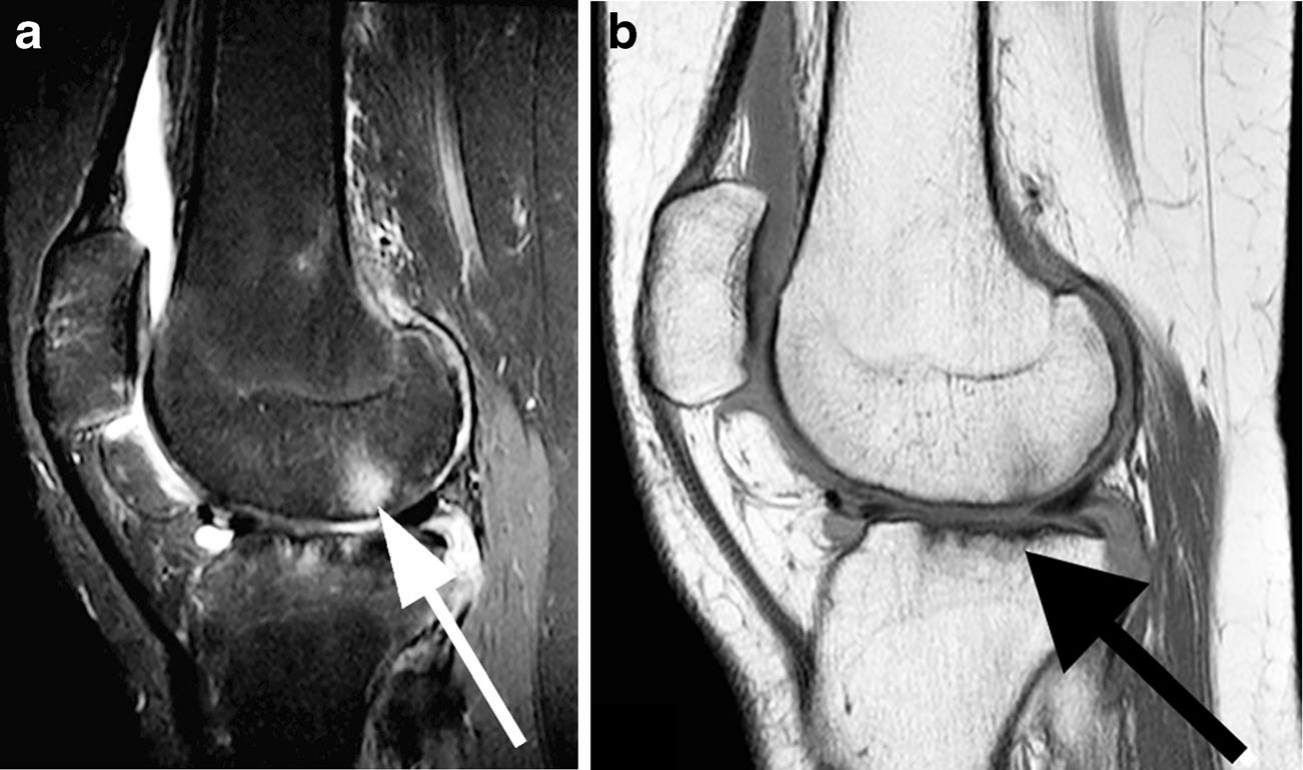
Sagittal MR images of the knee in a 16-year-old girl with poly-articular juvenile idiopathic arthritis. a T2-weighted sagittal MRI with fat suppression shows bone marrow oedema in the femur (*arrow*) and tibial plateau. b T1-weighted sagittal MRI shows bone erosions in the tibial plateau (*arrow*), with irregular signal intensity of the cortical bone and loss of the normal high signal intensity of trabecular bone
